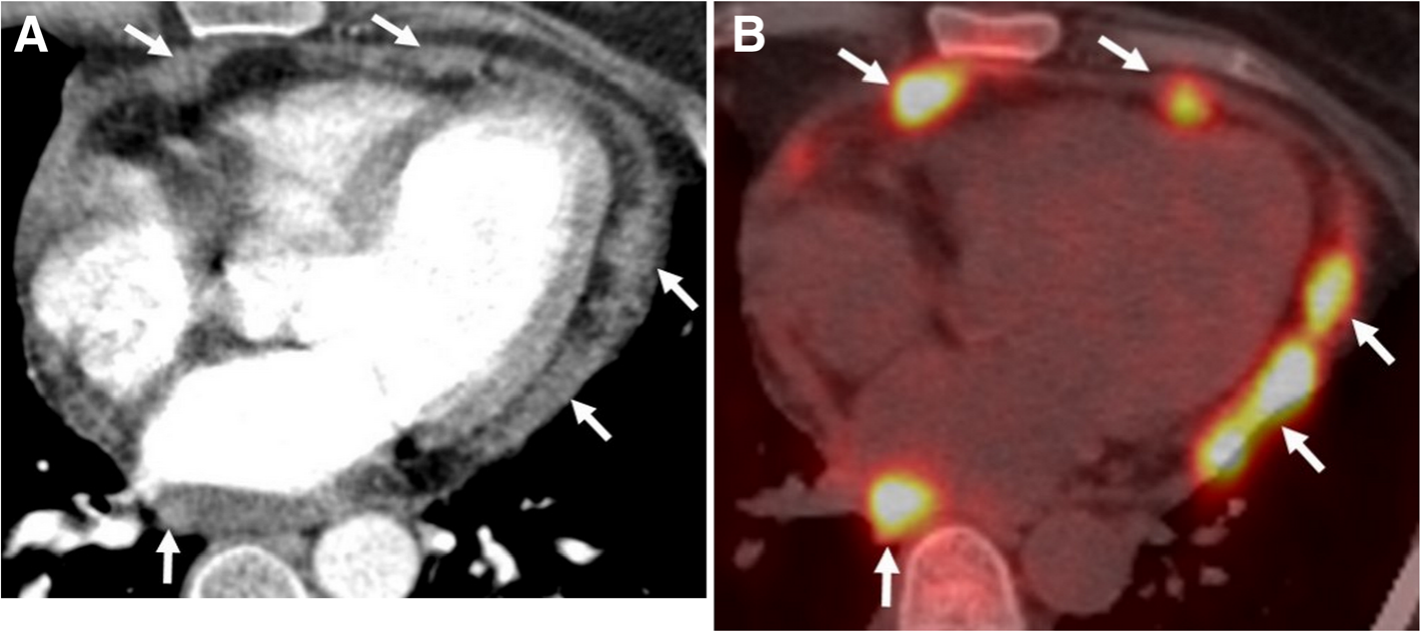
A 55-year-old woman with pathologically proven squamous cell carcinoma metastases from unknown origin. a Axial contrast-enhanced CT image demonstrates the nodular areas of pericardial thickening (arrows). b PET-CT scan reveals increased FDG uptake (arrows) at the same areas
